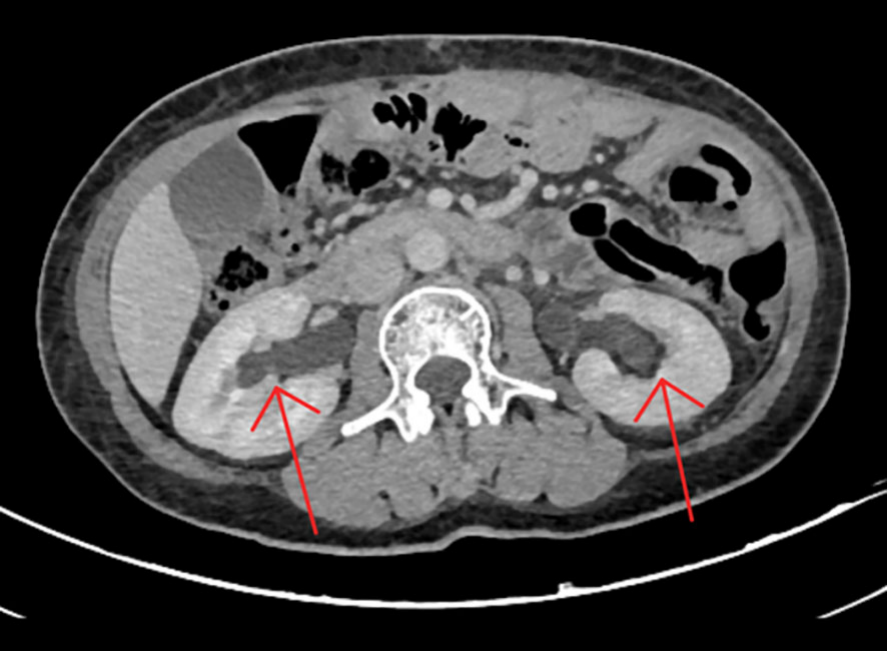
Non-contrast abdominal CT scan revealing minimal bilateral hydronephrosis. The scan showed mild dilation of the bilateral renal pelvis and collecting systems, without visible ureteral stones, wall thickening, strictures, or other obstructive lesions. These subtle findings likely contributed to the initial underestimation of the severity of urinary tract obstruction.
